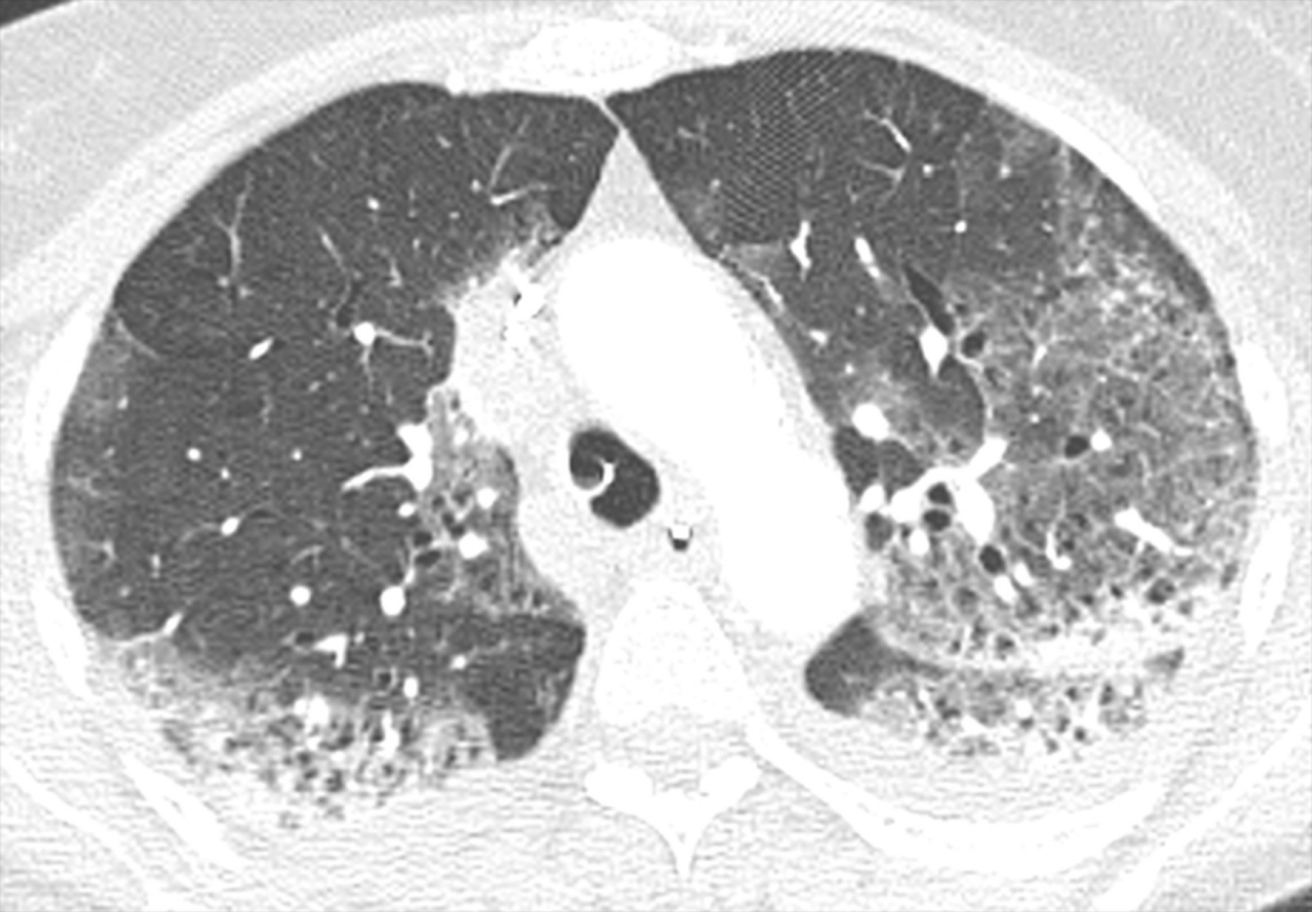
Crazy paving. Axial reconstruction of a CT in lung window demonstrating crazy paving.
